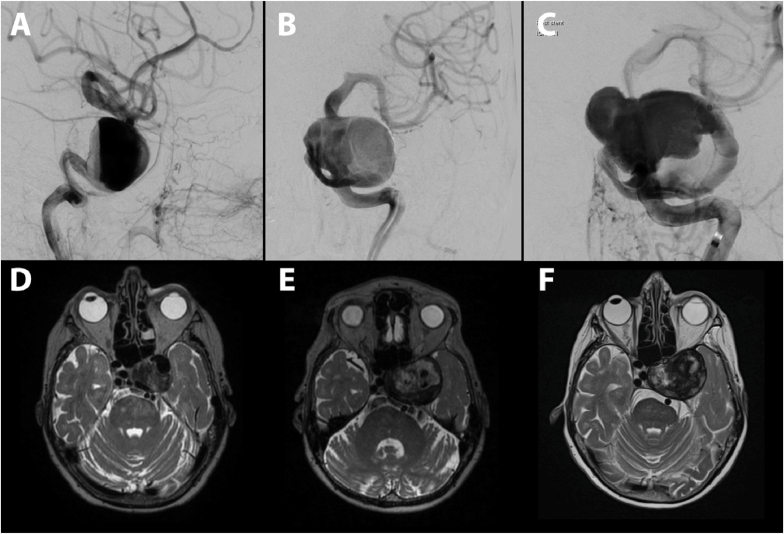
Case 11. Failed occlusion of a giant left Internal Carotid artery (ICA) aneurysm. Lateral (A) and AP (B) diagnostic angiograms of the left ICA. AP angiogram immediately after deploying the flow diverter. Initial (D), Interim (E) and Final MRI- T2 axial views of the patient demonstrating the increasing size of the aneurysm following failed occlusion.
